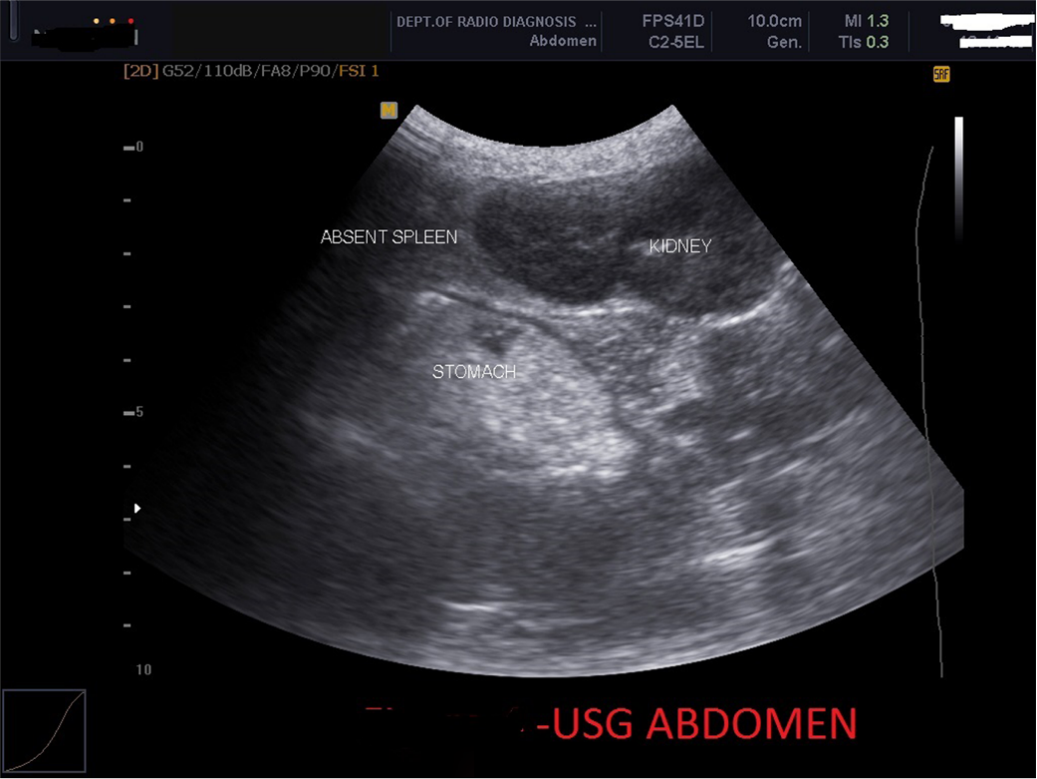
Abdominal ultrasound showing absent spleen.
